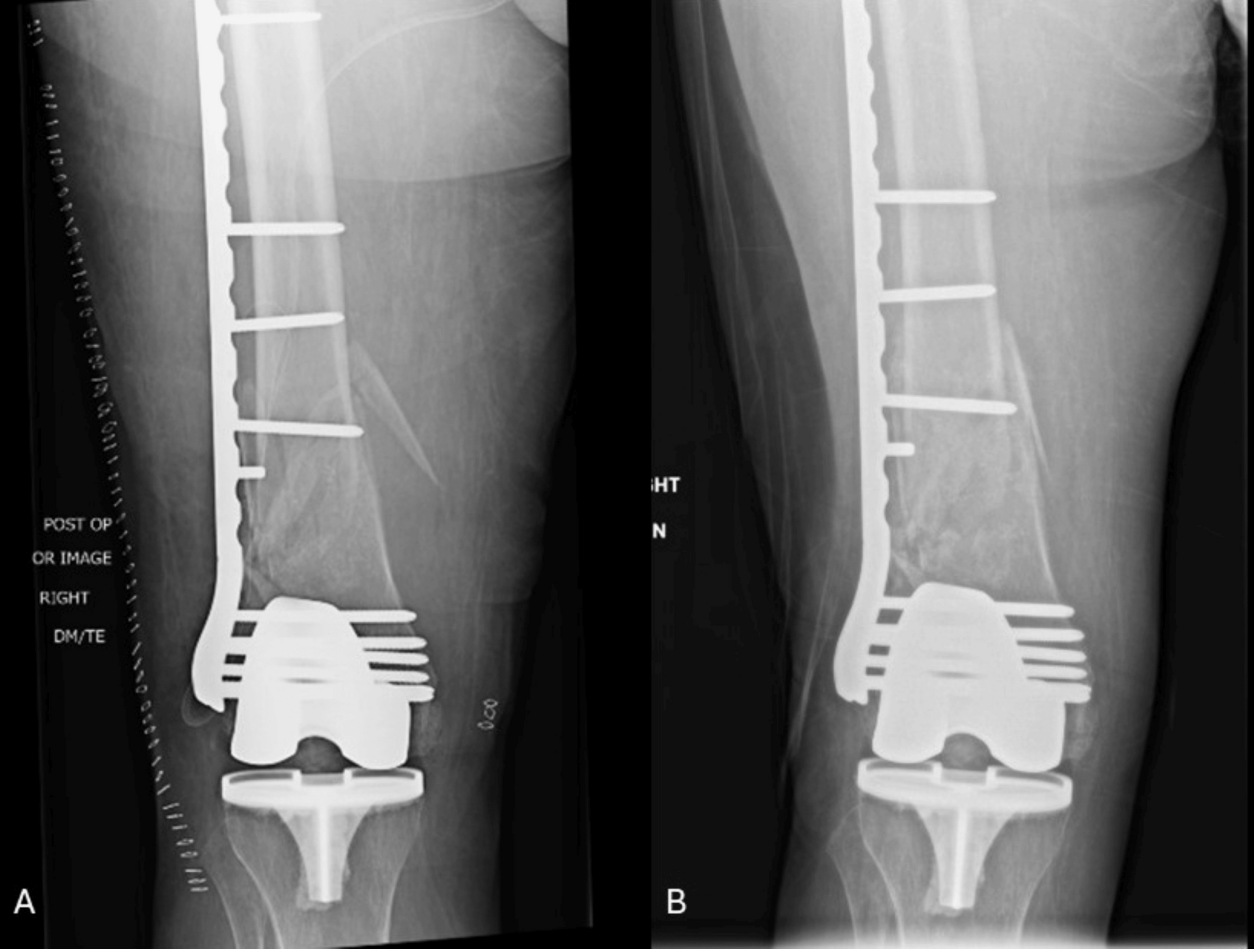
Postoperative and follow-up radiographs Immediate postoperative AP of the distal femur (A) and 4.5-month follow-up (B) demonstrating radiographic signs of healing and a lateral distal femoral angle of 82.9°.
